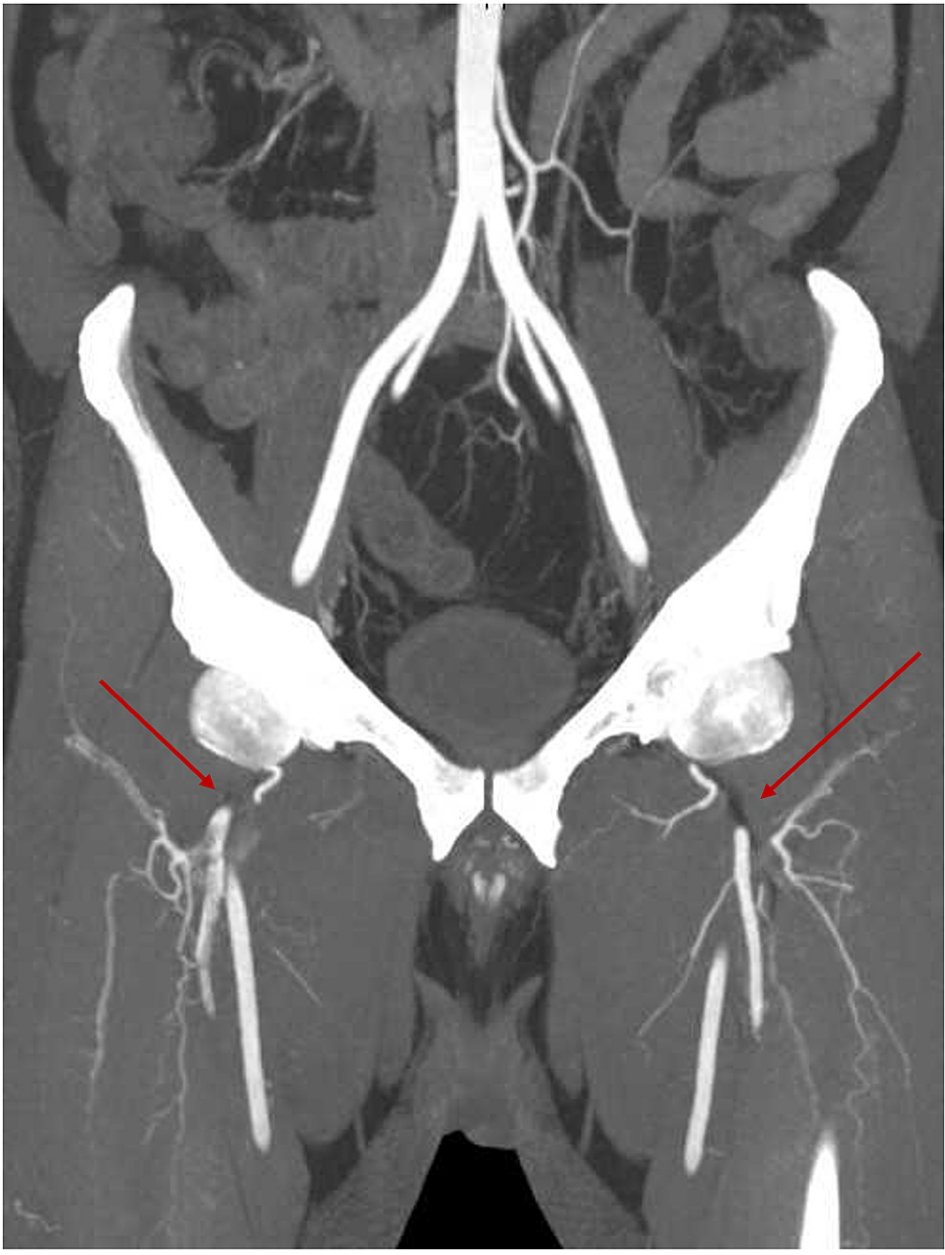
Computed tomography aortogram maximum-intensity projection showing filling defect within the profunda femoris artery bilaterally with a faint rim of contrast surrounding starting just distal to its origin and extending inferiorly as it gets its perforator branches
